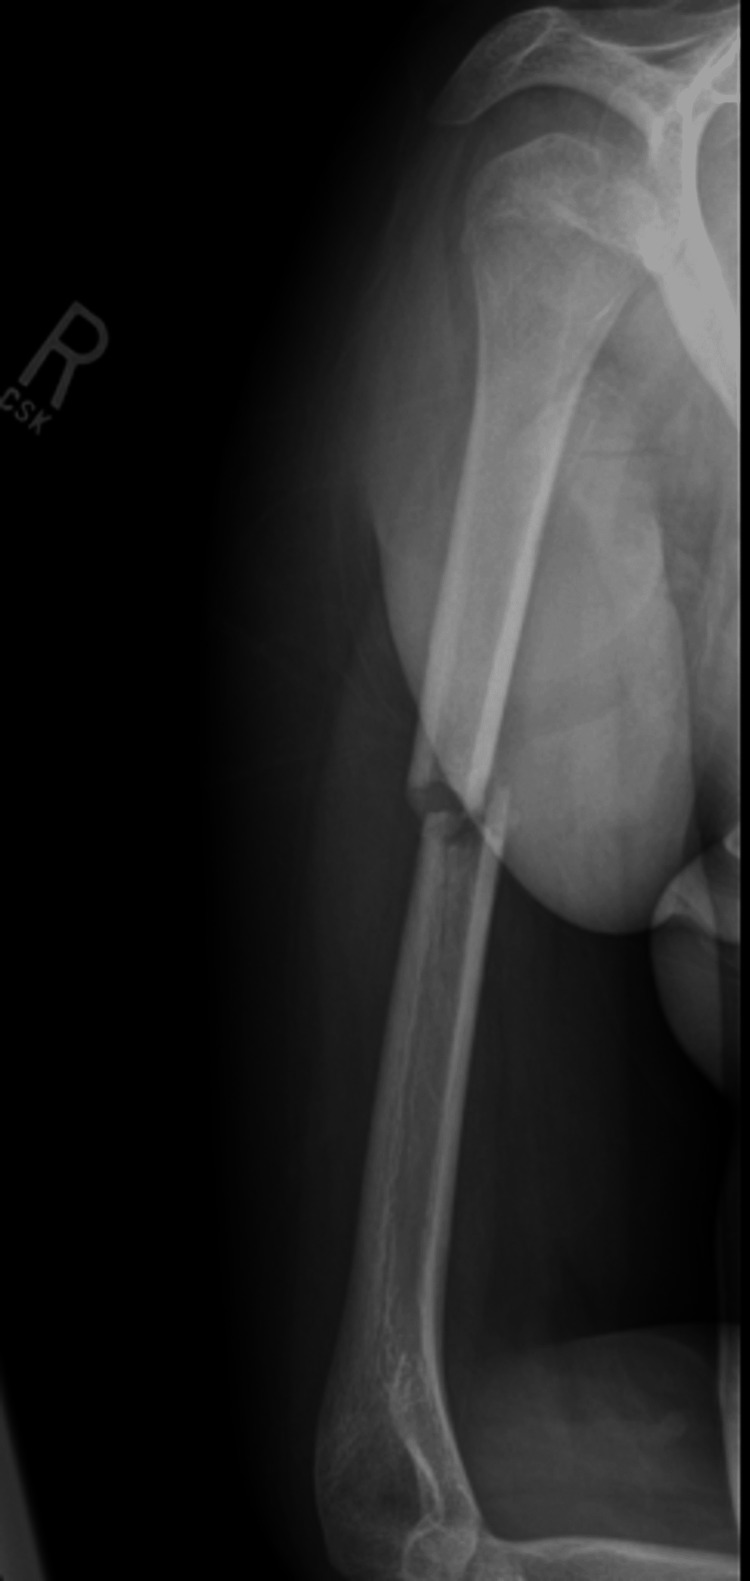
Radiograph of the humerus at 11 weeks post-injury. No callus formation noted. No bone overgrowth or hypertrophy of the fracture site noted.
